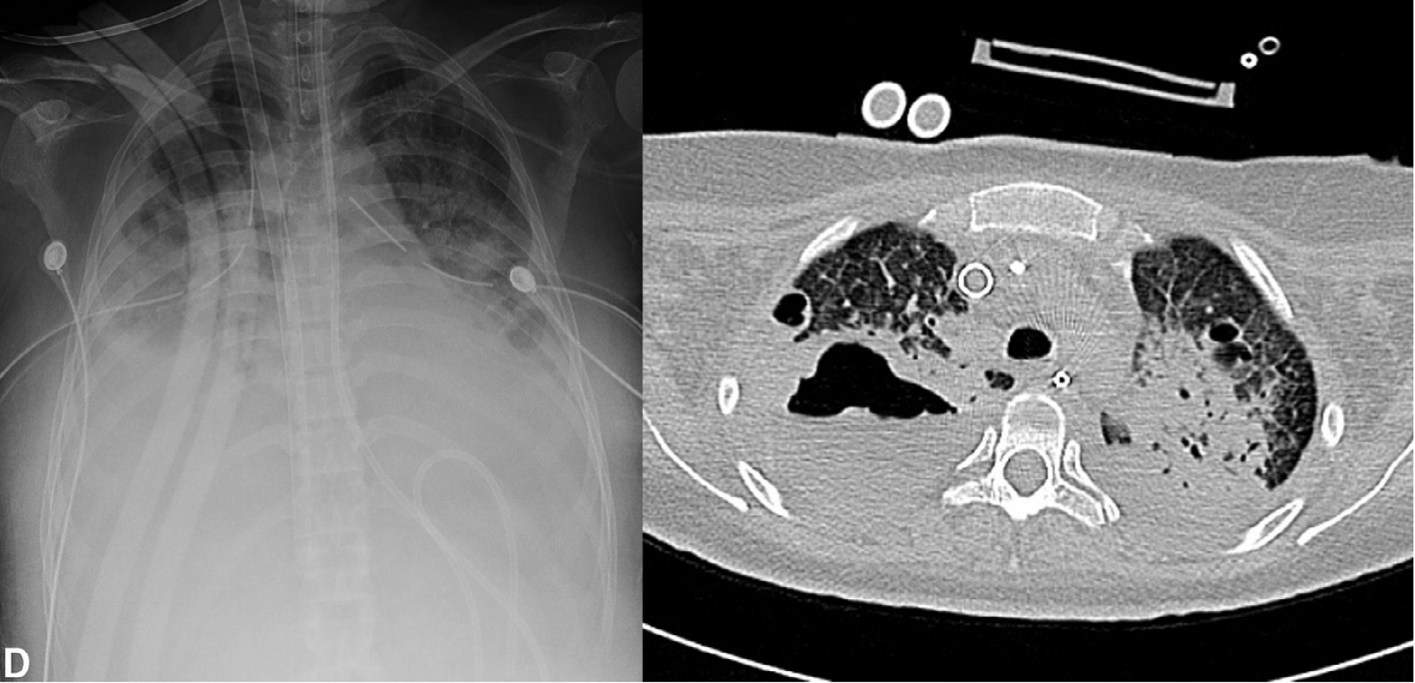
Patient’s lung contusion at the initial evaluation. Thorax anteroposterior radiographs and thoracic CT showing extensive lung contusion.
